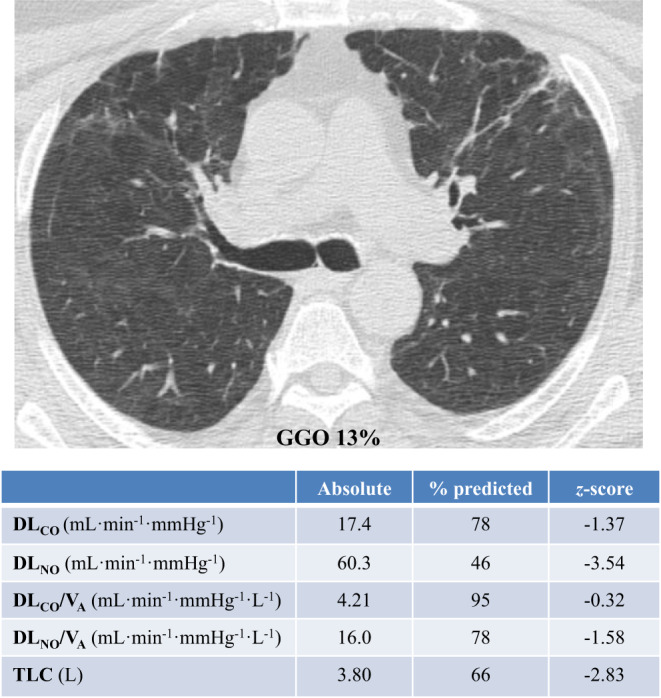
Axial CT scan acquired at the bifurcation of main bronchi (carina) in supine position in a representative subject who had severe COVID‐19 pneumonia treated by invasive mechanical ventilation. Note the discrepancy between DL_NO_ and standard DL_CO_ in the presence of moderate GGO extent. Abbreviations as in Table 1
